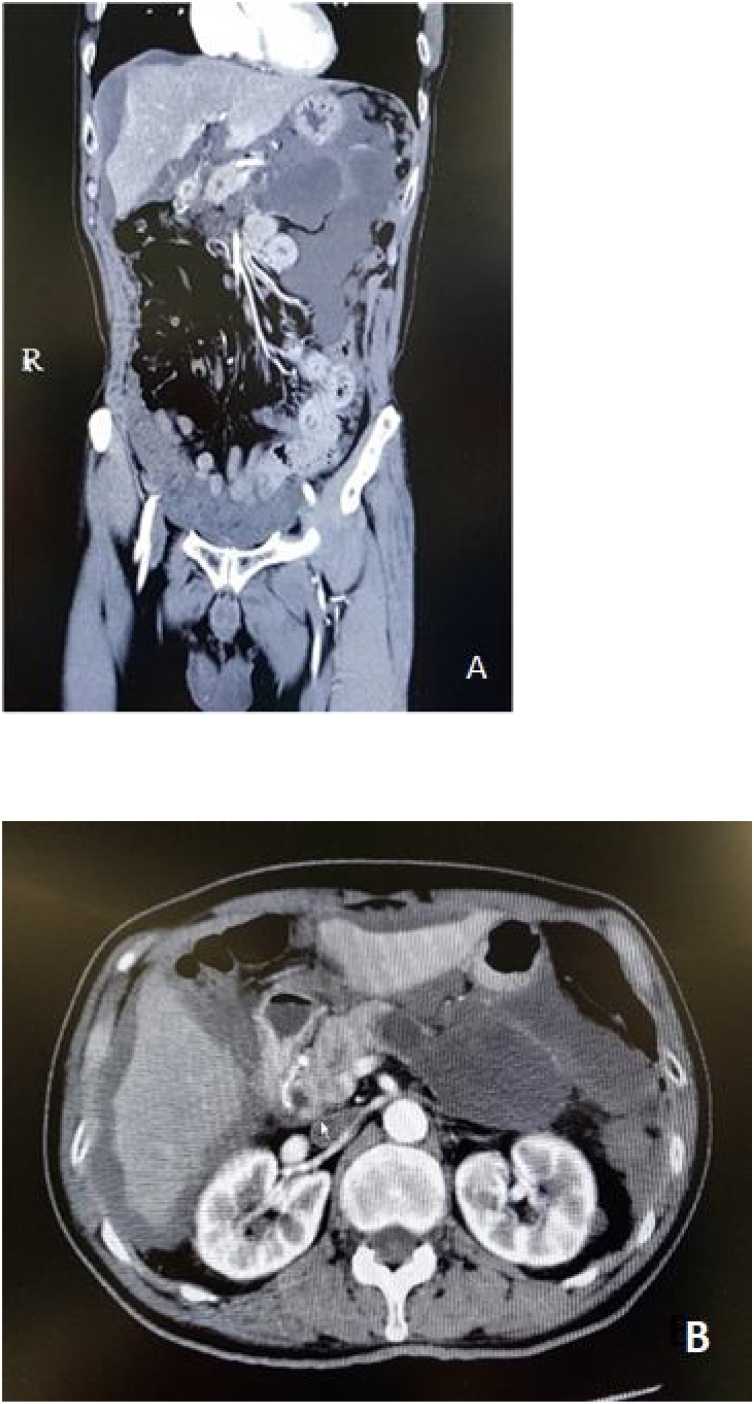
CT scan image showing cystic lesion at tail of pancreas and mucinous ascites (A) Coronal section show mucinous content around pancreatic cyst and upper abdominal part (B) Axial section show scallop liver.
